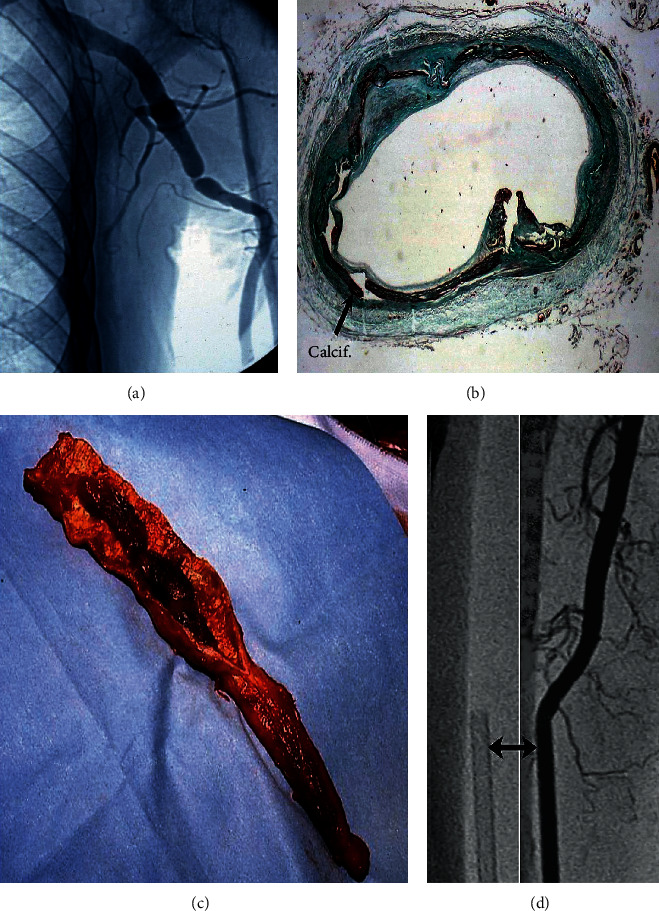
(a) Asymptomatic brachial artery occlusion fortuitously discovered on a postoperative coronary angiogram in a patient having undergone radial artery grafting. This case belongs to the early series. Nowadays, preoperative echo-Doppler of the upper-limb would have revealed the lesion, and RA would not have been used. (b, c) Media calcinosis of the radial artery. (b) Nonobstructive mediacalcinosis in a diabetic patient; (c) atheromatous occlusion with intraluminal thrombus. (d) Traumatic injury for transradial artery coronary intervention. Note that the size of the sheath (arrow) equals that of the vessel lumen.
